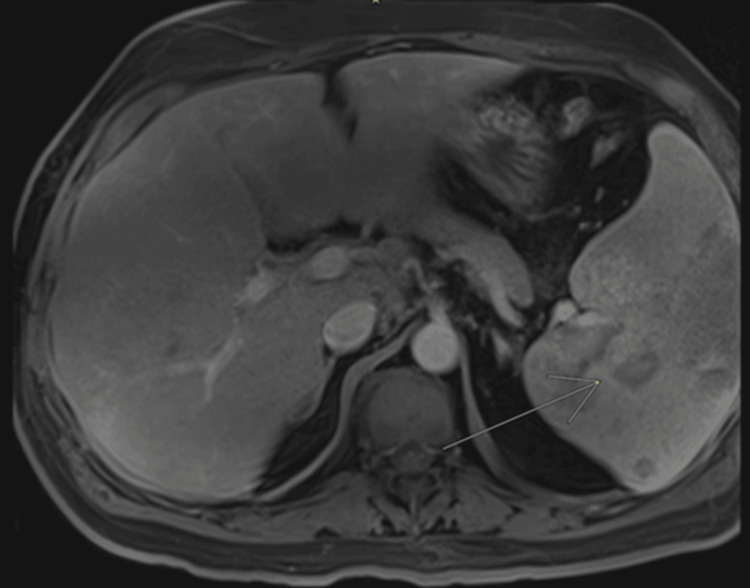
T1-weighted magnetic resonance imaging (MRI) with paramagnetic contrast enhancement. The splenic parenchyma exhibits multiple rounded lesions (yellow arrow) visualized after contrast administration. These lesions are predominantly hypointense at the periphery, featuring diffuse central nodular enhancement with variable diameters measuring up to 38mm. Image suggestive of an infiltrative process.
